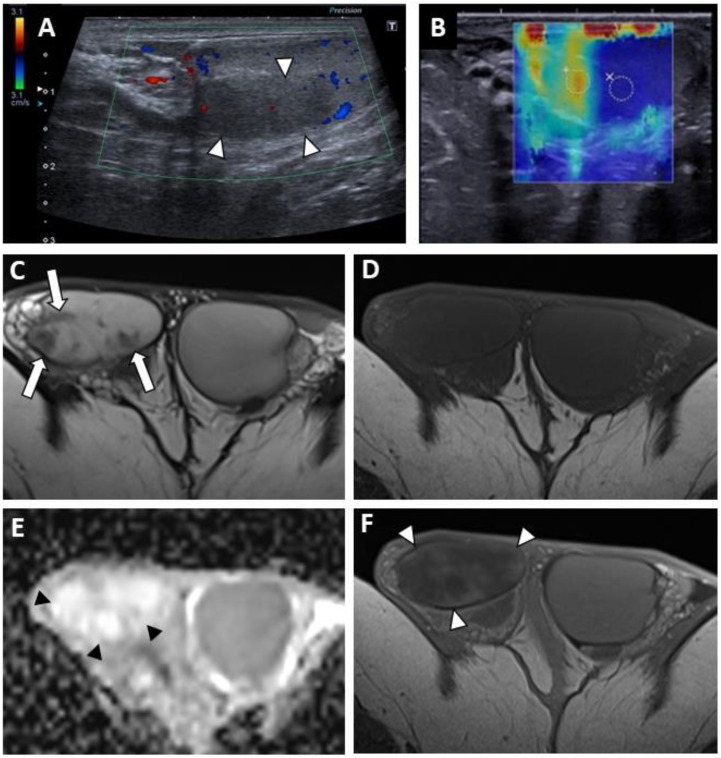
BOTT of the right testis in a 40-year-old man (symptomatic retroperitoneal nodes). Longitudinal image of US on Colour Doppler mode showing poorly circumscribed hypoechoic and hypovascularised nodular areas (white arrowheads) (A). Shear-wave elastography mapping showed a focal induration (B) (different slice plane from image (A)). Axial T2W image showed multiple irregular areas with a hypo-intense signal (white arrows) (C). Axial T1W image without a detectable focal lesion (D). ADC map of the diffusion sequence demonstrated a focal area with an elevated ADC value compared with the contralateral testis (black arrowheads) (E). After the intravenous injection of gadolinium chelate, confluent areas with reduced enhancement were visible (note that these areas are overlapping the nodules visualized in T2) (F).
